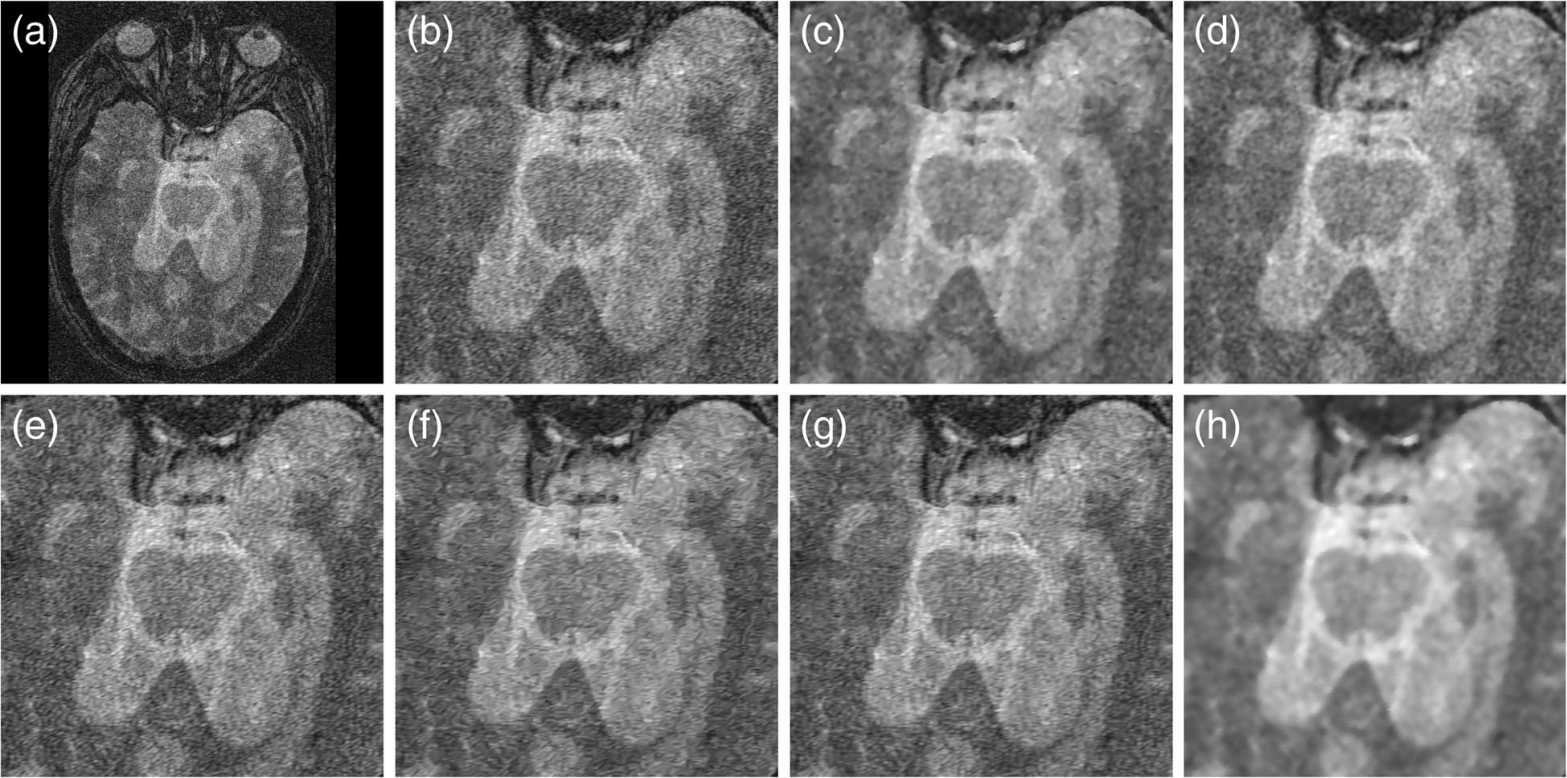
Comparison of the restoration of noisy MR images with low resolution. a Input MR image. b Input image in magnified view. c IBF. d ADF. e LMMSE. f NLM. g BM3D. h Proposed
